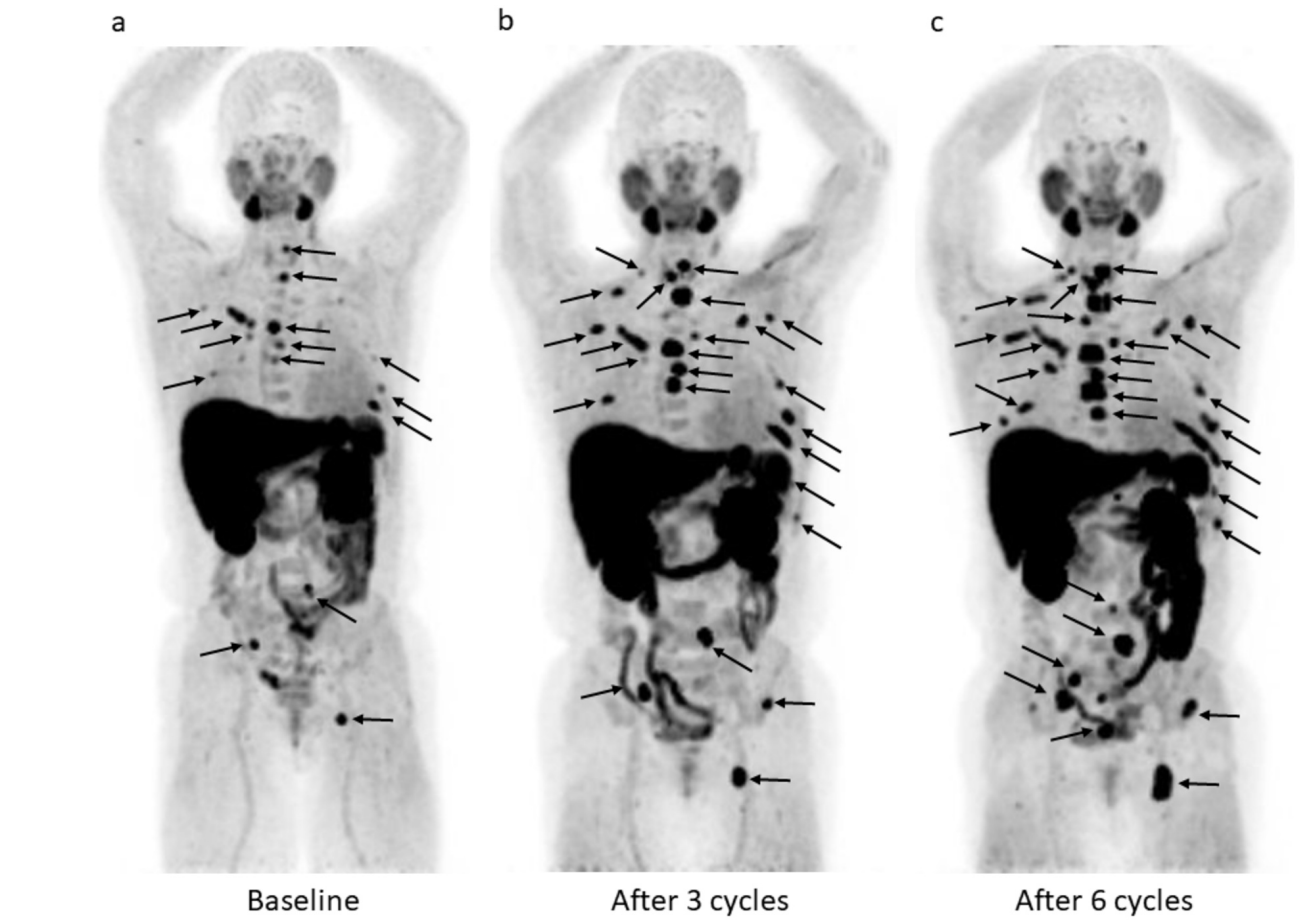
A 59-year-old man with bone metastatic castration-resistant prostate cancer. Maximum intensity projection (MIP) images in the coronal projection of sequential ^11^C-choline PET/CT scans carried out at (a) baseline, (b) after three cycles, and (c) after six cycles of Ra-223 therapy. (a) Pretreatment MIP image showing multiple areas of increased focal activity in the spine, ribs, and the pelvic bone (arrows). (b) MIP image after three cycles showing increasing tumor activity in the existing lesions and the new uptake spots of spine, ribs, and pelvic bone (arrows). (c) Post-treatment MIP image showing further progression with new lesions (spine, ribs, and pelvic bone) (arrows).
